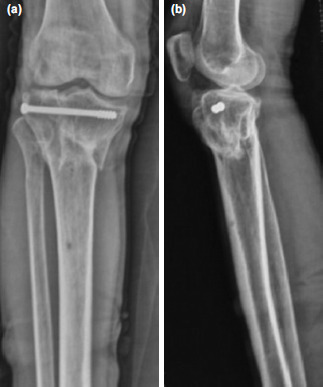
Case No.12, radiographs at final follow up showing union. (a) Antero-posterior view. (b) Lateral view.
